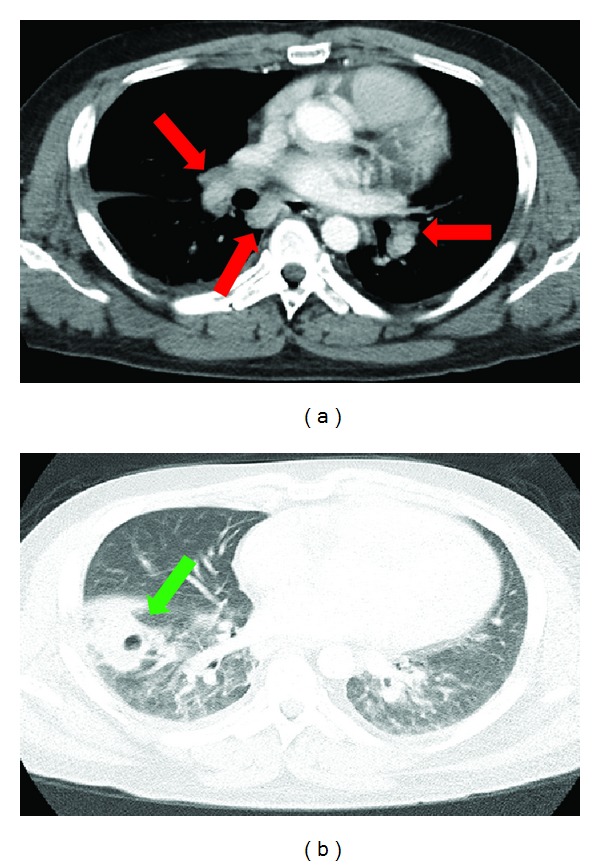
Axial contrast-enhanced CT of the chest demonstrates bilateral soft tissue densities (red arrows) consistent with bilateral hilar adenopathy (a). Opacity in right lower lobe with air-fluid level (green arrow) is consistent with pulmonary abscess (b).
